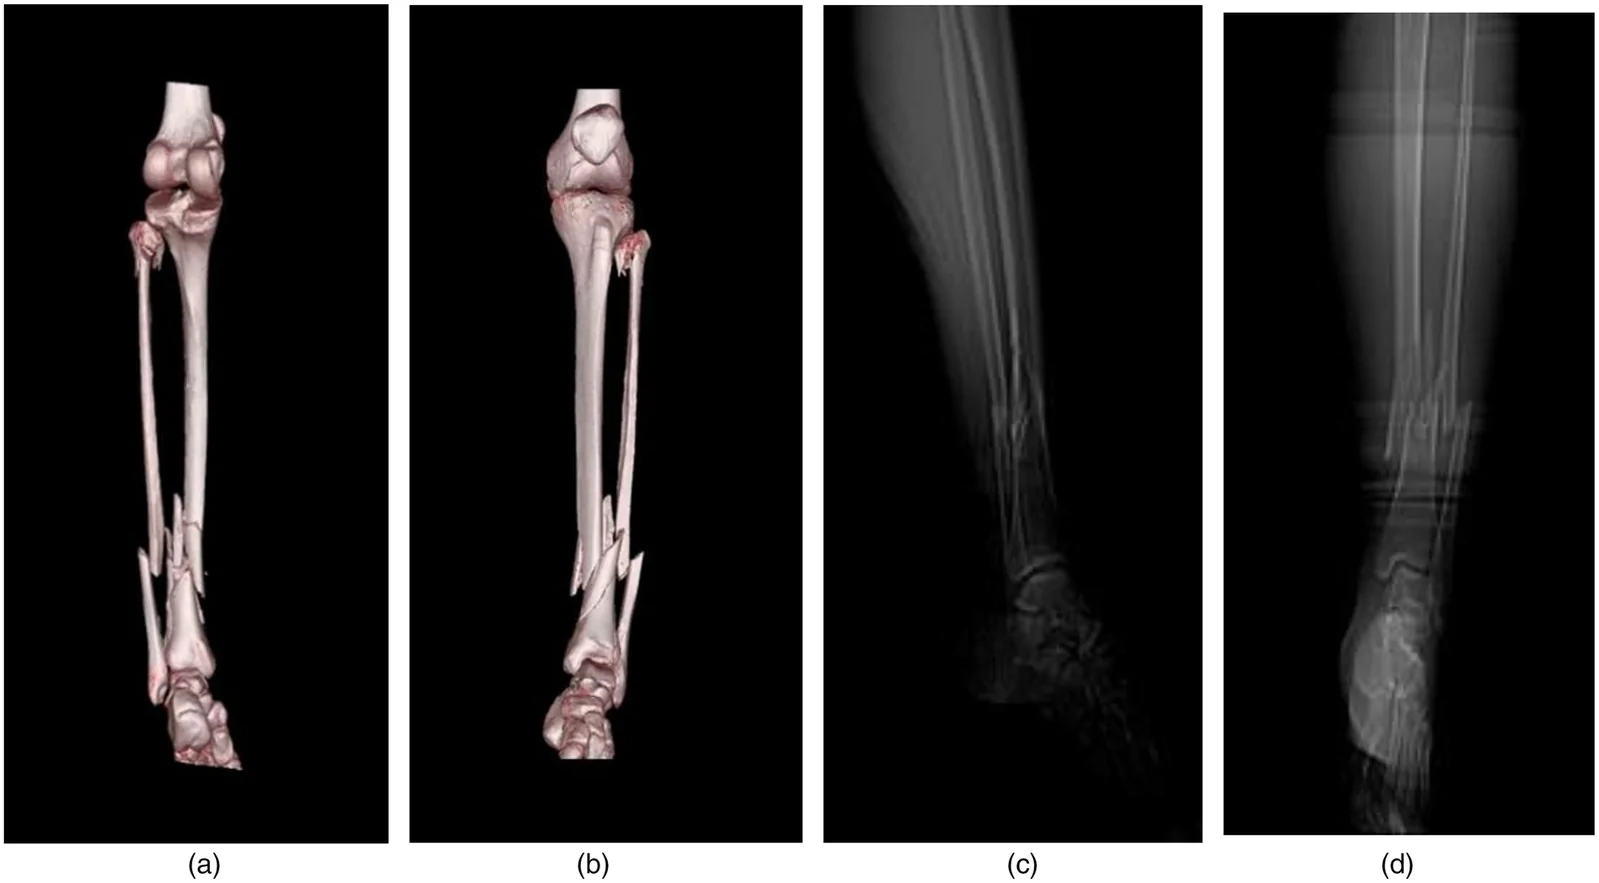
(a–d) on CT scans taken after admission, a comminuted fracture of the distal tibia is visible.
